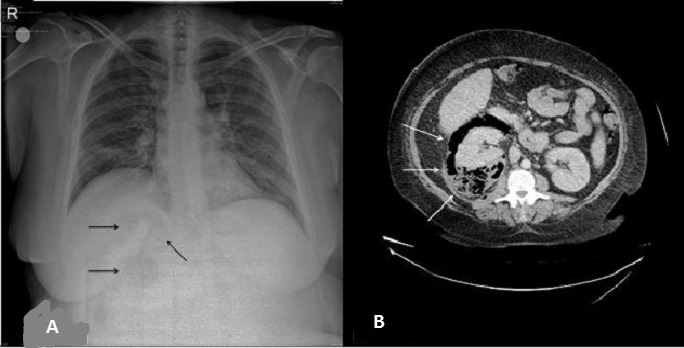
(A) abdominal X-ray and computed tomography showing emphysematous pyelonephritis; (B) ultrasonography shows echogenic foci with ’'dirty’’ shadowing within the kidneys; on CT the collections of gas are seen directly as hypoattenuating foci in the renal lodge
